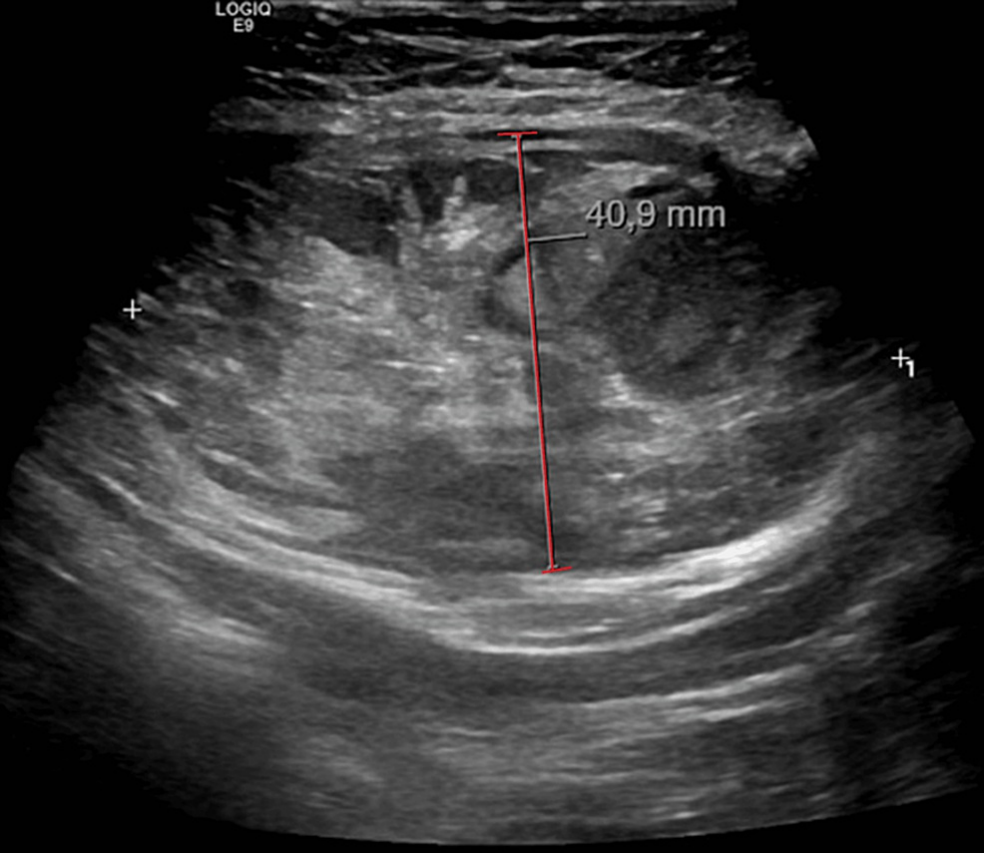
Heterogeneous collection (4x7cm) in the abdominis rectus muscle topography on ultrasound compatible with rectus sheath hematoma.
